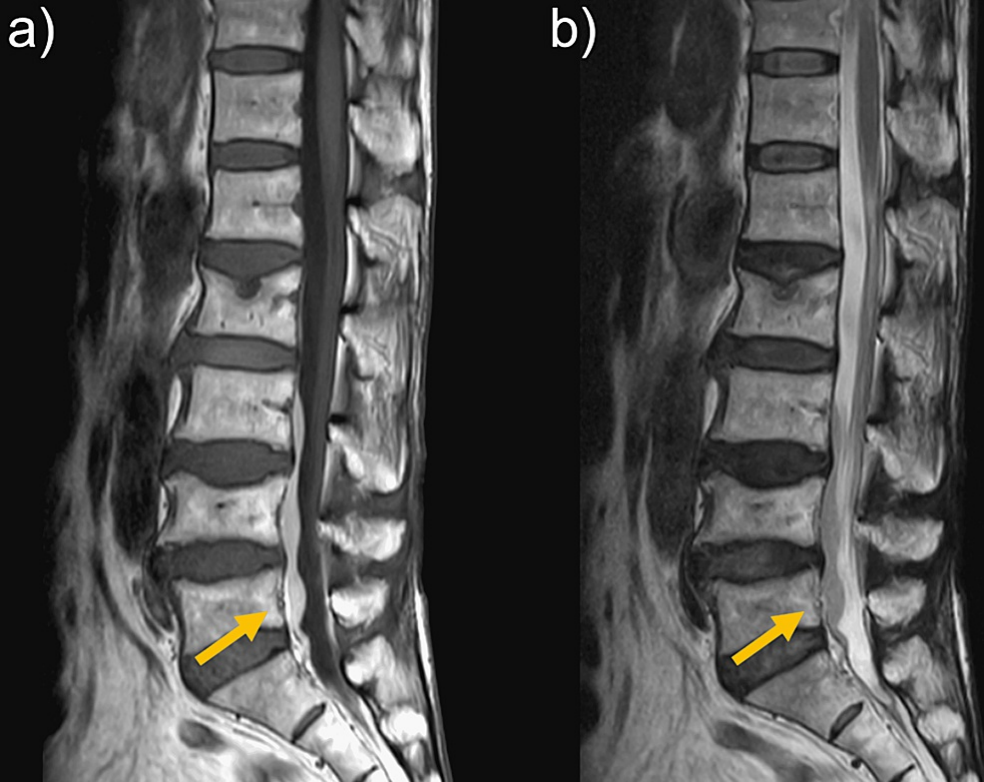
Lumber MRI at seven days after onset (a) A hematoma-like mass with a high signal on T1WI. (b) An isointense signal on T2WI.
